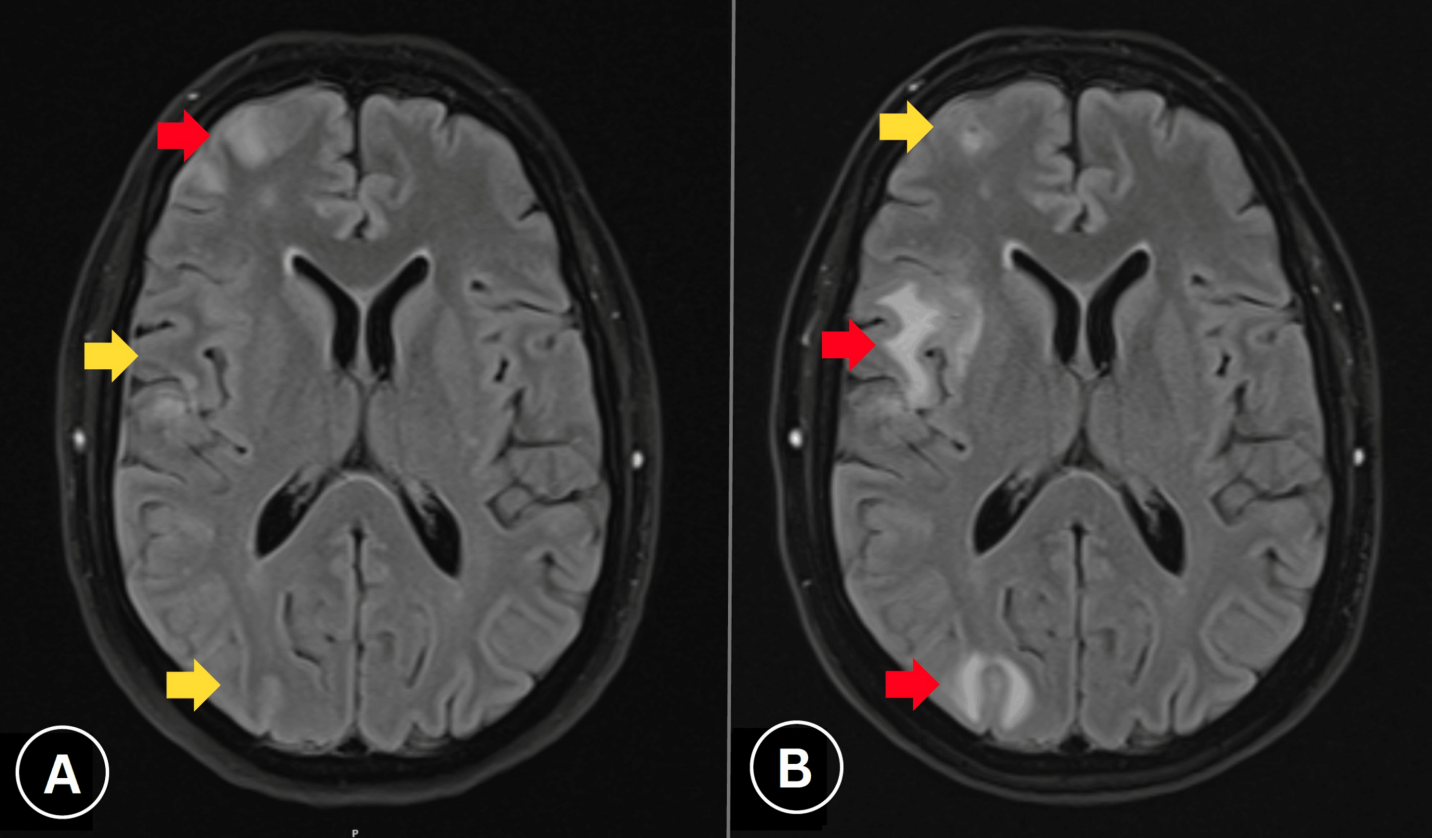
Serial MRI demonstrating lesion evolution. (A) Baseline MRI showing right hemispheric cortical lesion distribution. (B) Two-week follow-up demonstrates progression in parietal-occipital regions (red arrows) with concurrent resolution of frontal enhancement (yellow arrows), consistent with inflammatory rather than neoplastic aetiology.
